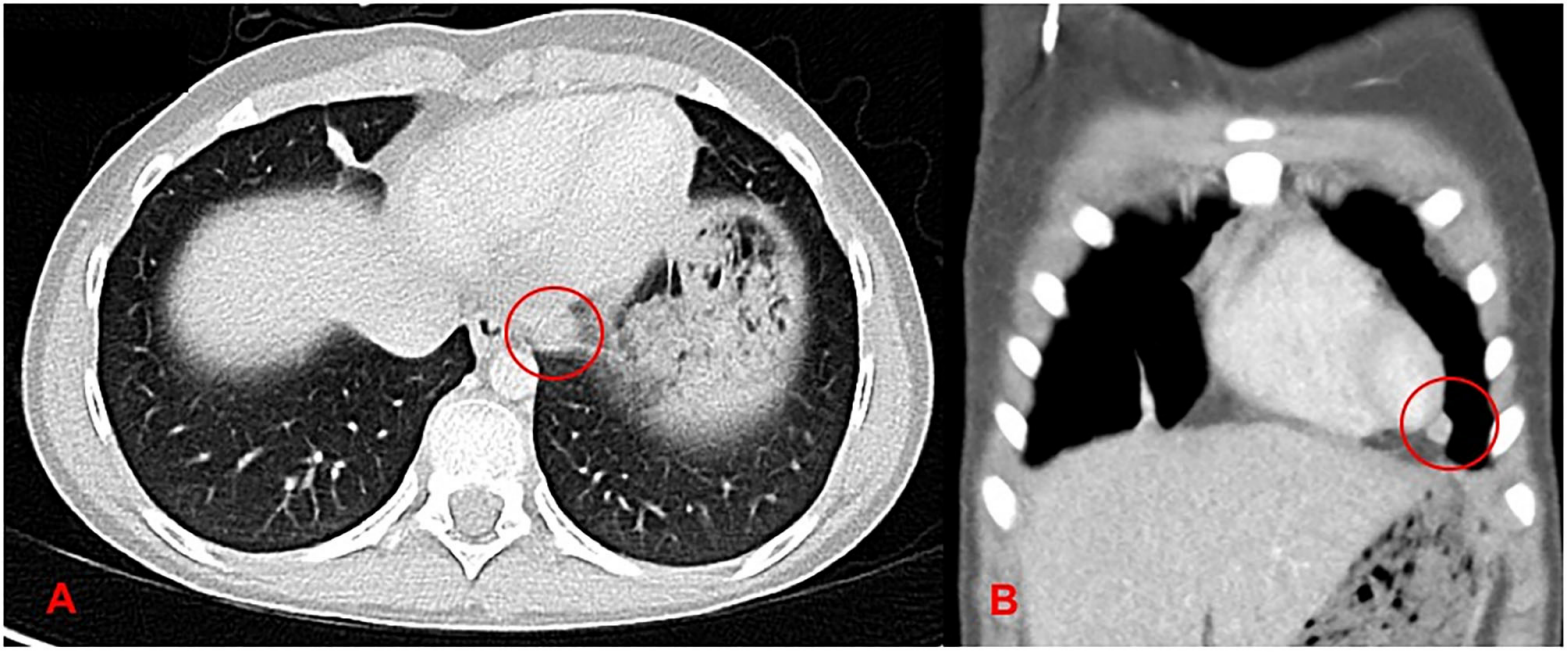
Preoperative CT revealing multiple metastases: (A) shows a paraesophageal metastasis (red circle) and (B) demonstrates a pericardial metastasis (red circle). Both were removed surgically during initial resection (Patient #13).
